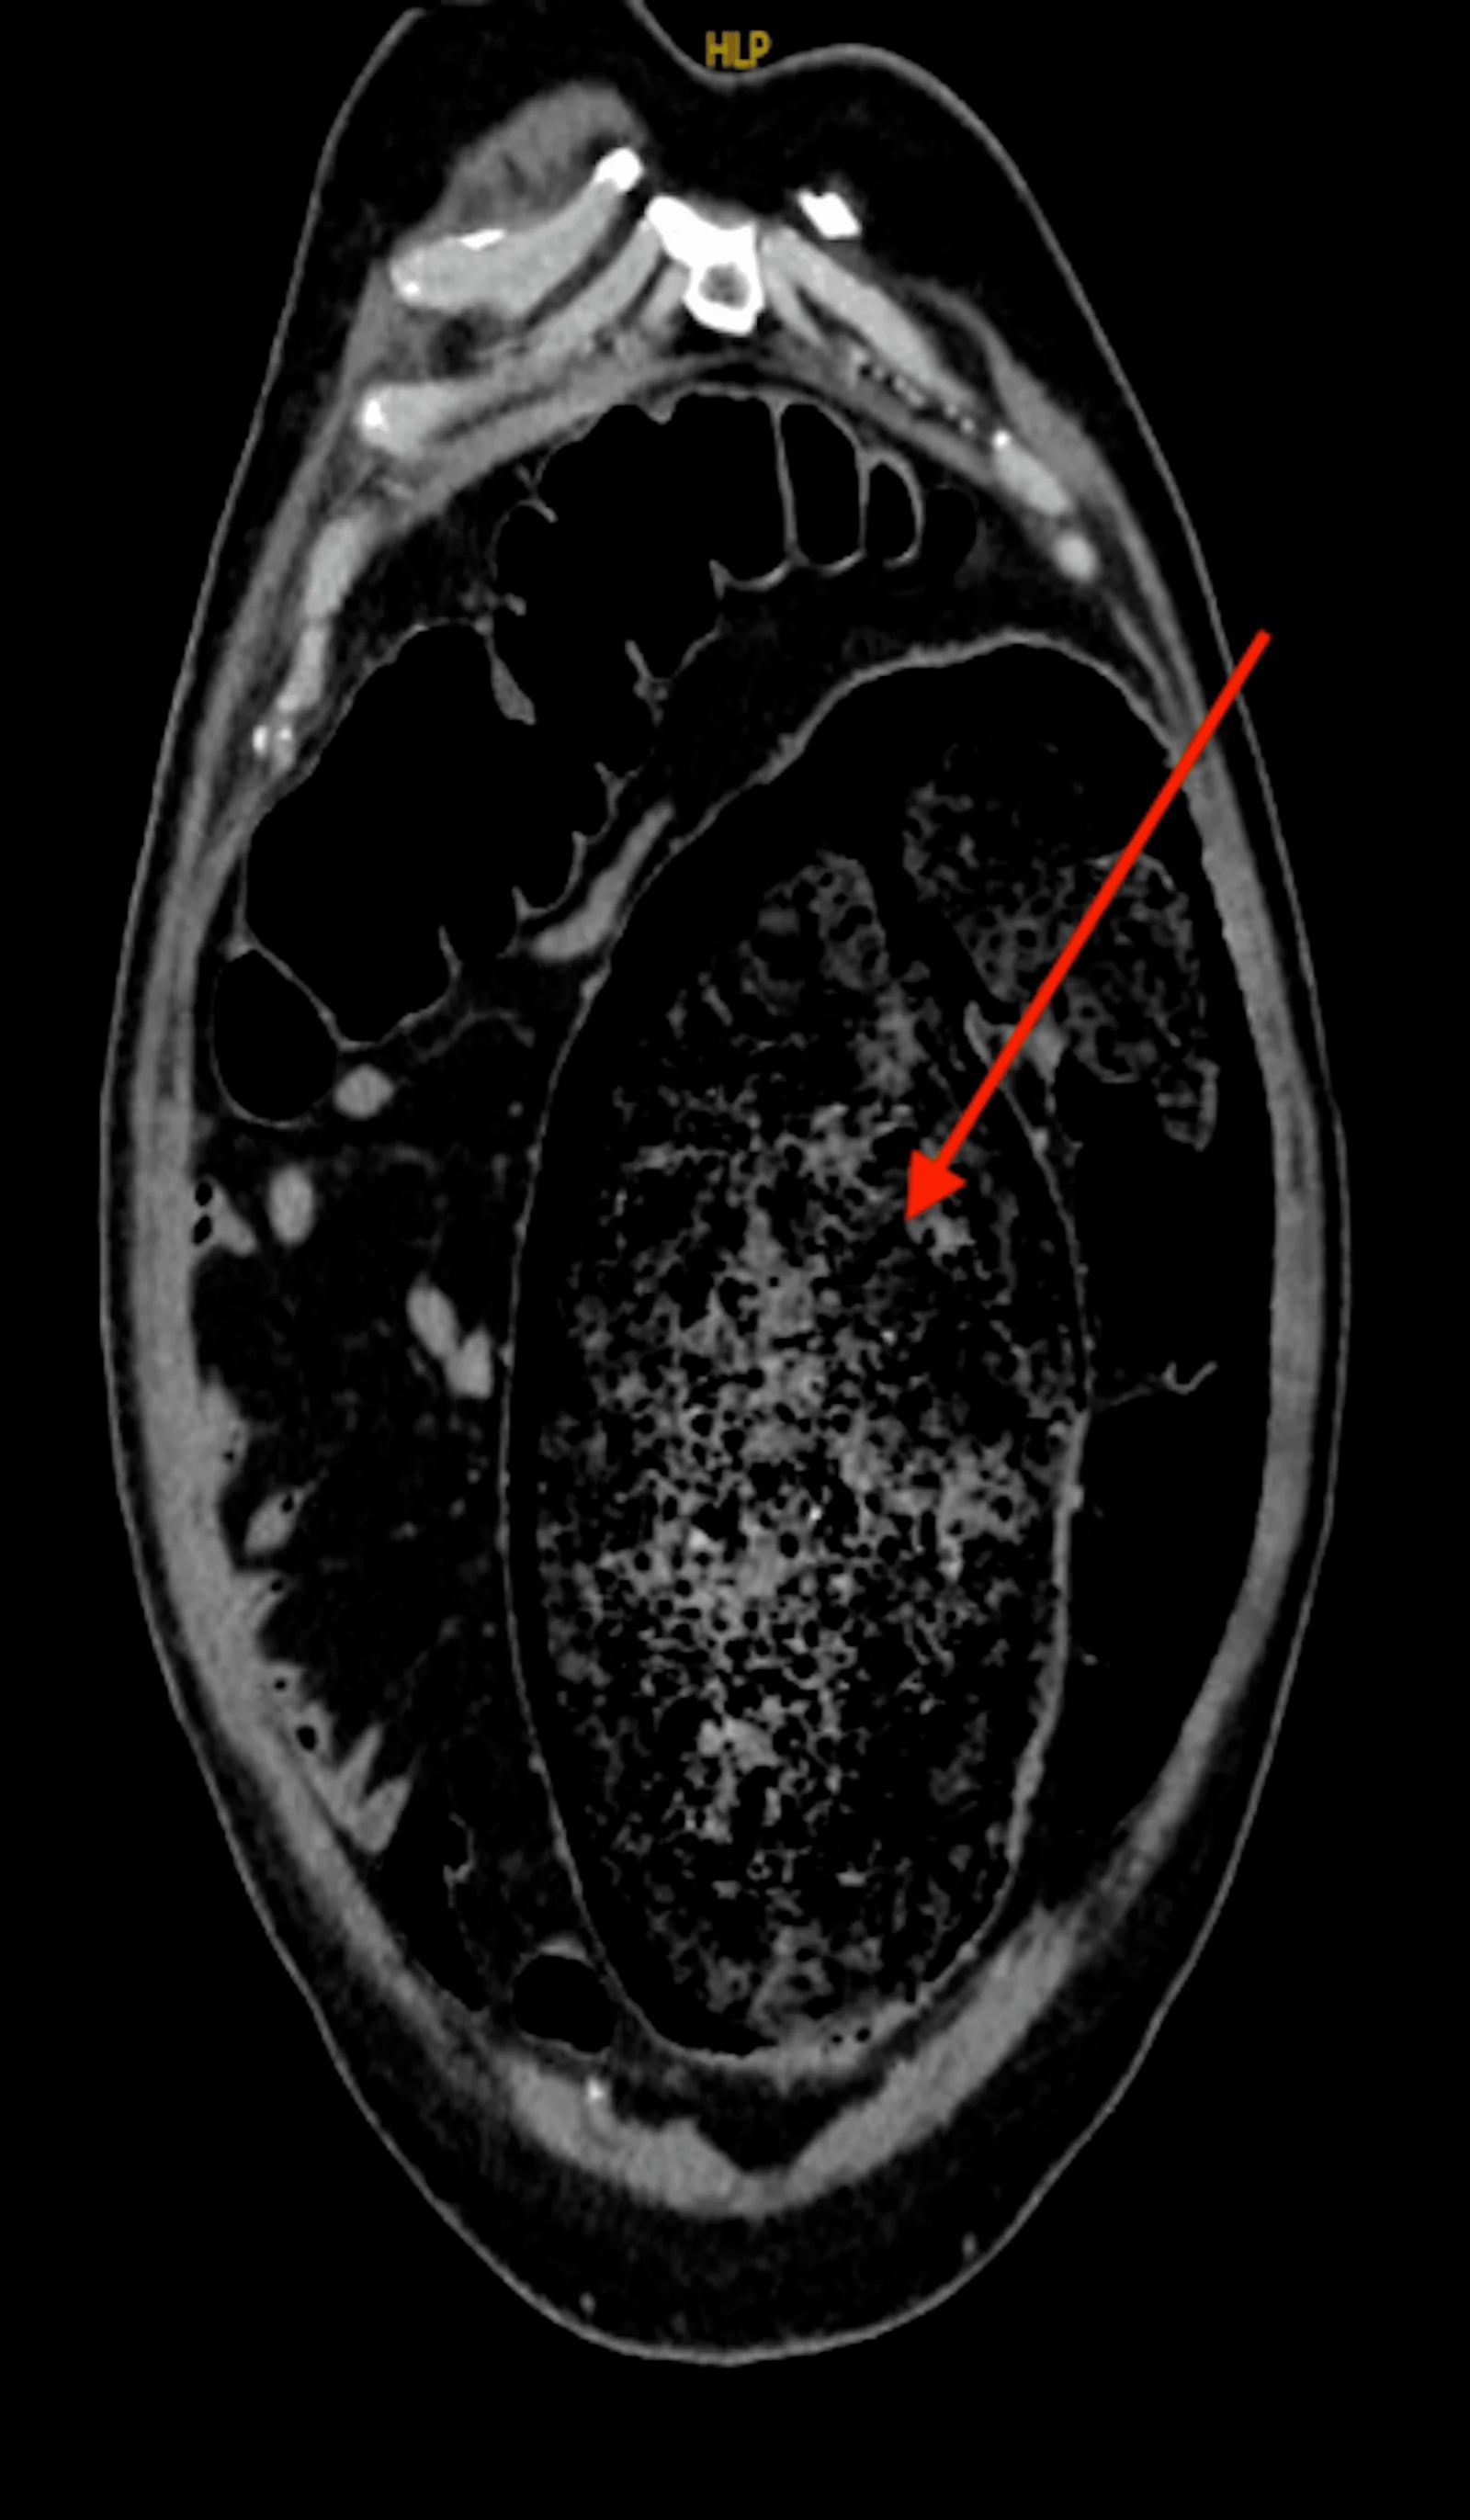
Axial contrast-enhanced compted tomography (CT) abdomen CT abdomen showing a large sigmoid fecaloma (red arrow) causing marked colonic dilatation and ileus, with associated features suggestive of stercoral colitis in a patient with severe hypothyroidism-induced gastrointestinal hypomotility.
